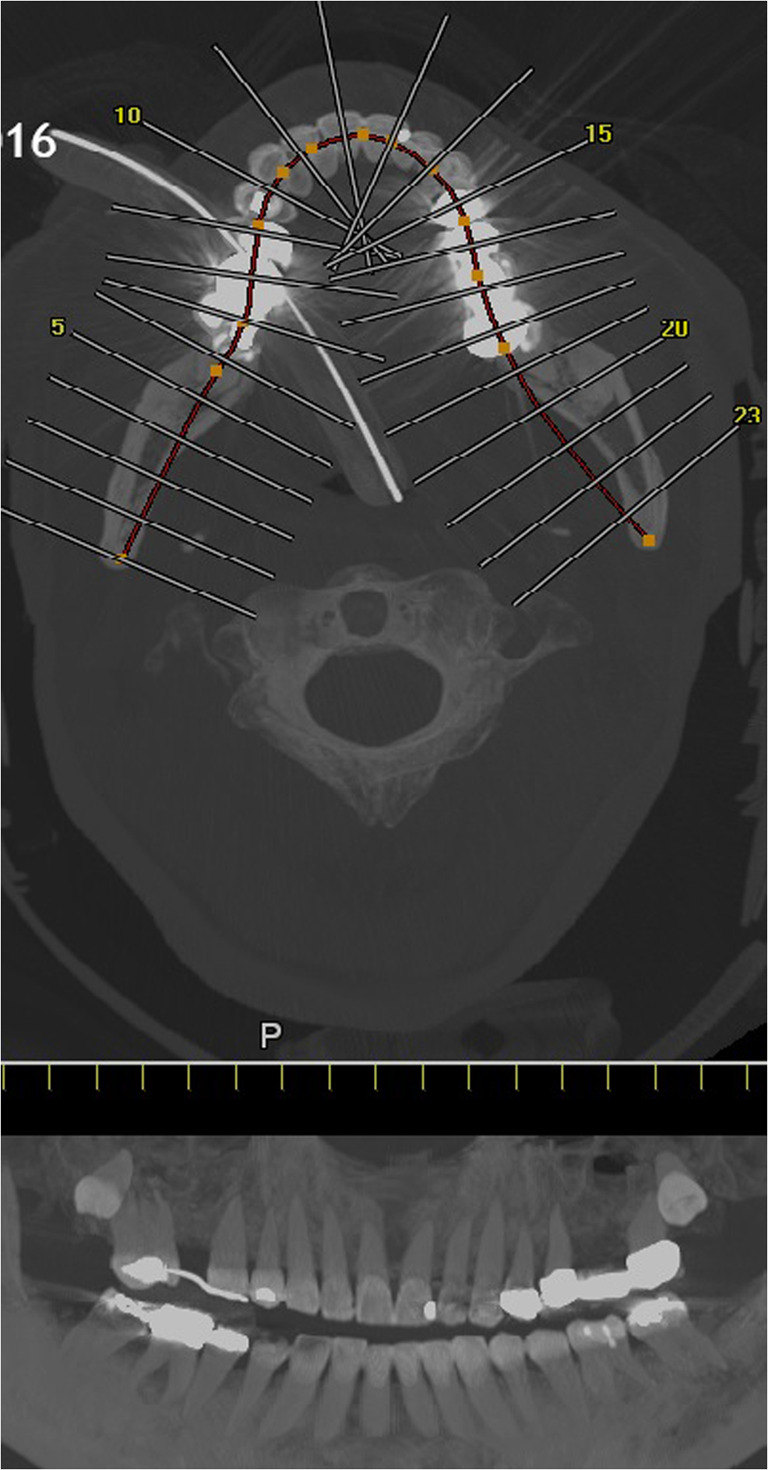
Dental reconstruction for identification purposes according to post-mortem computed tomography data
